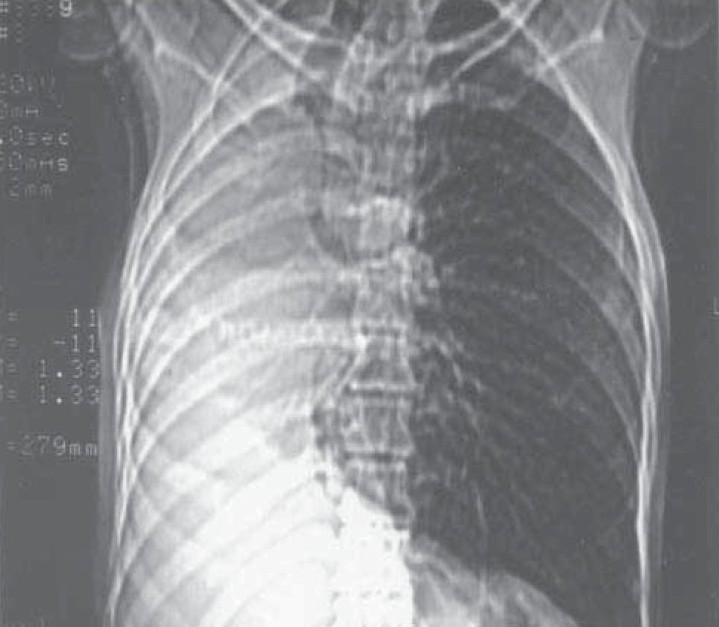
CT Scannogram showing right side opaque hemi thorax with signs of volume loss.
